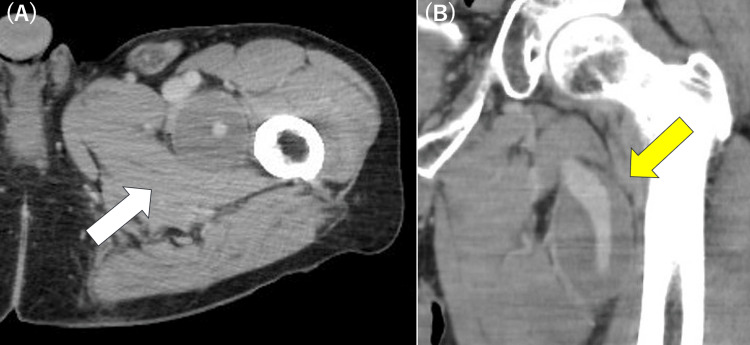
Preoperative CECT images (A) Axial view showing a deep femoral artery aneurysm (white arrow). (B) Coronal view demonstrating the aneurysm (yellow arrow), with a maximum longitudinal diameter of 69 mm. CECT: contrast-enhanced computed tomography
